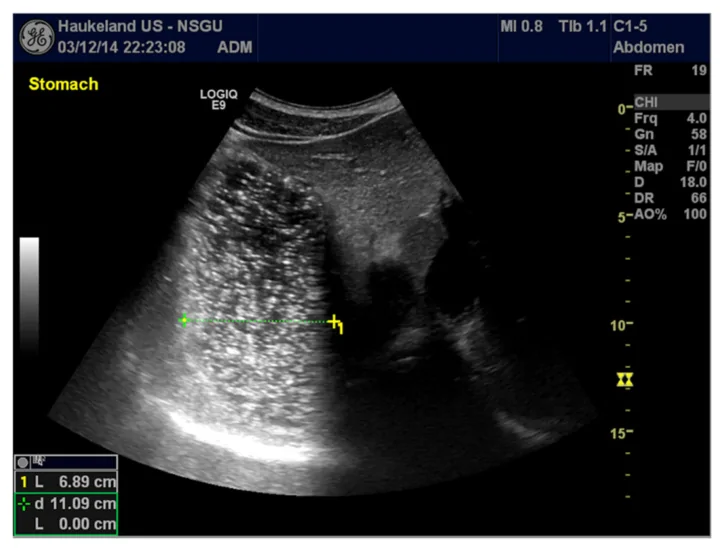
A 2D ultrasonographic image showing the proximal part of the stomach. The second measurement is obtained in an oblique frontal plane, in which the gastric diameter is measured using the hemidiaphragm as an anatomical landmark.
